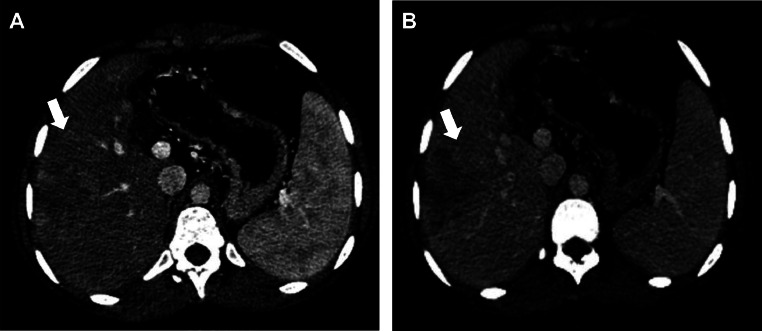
Axial CT scan showing a multinodular infiltrative HCC in segments VI and VII; hypervascular lesions on arterial phase (A) with washout in portal phase (B) (white arrow).
